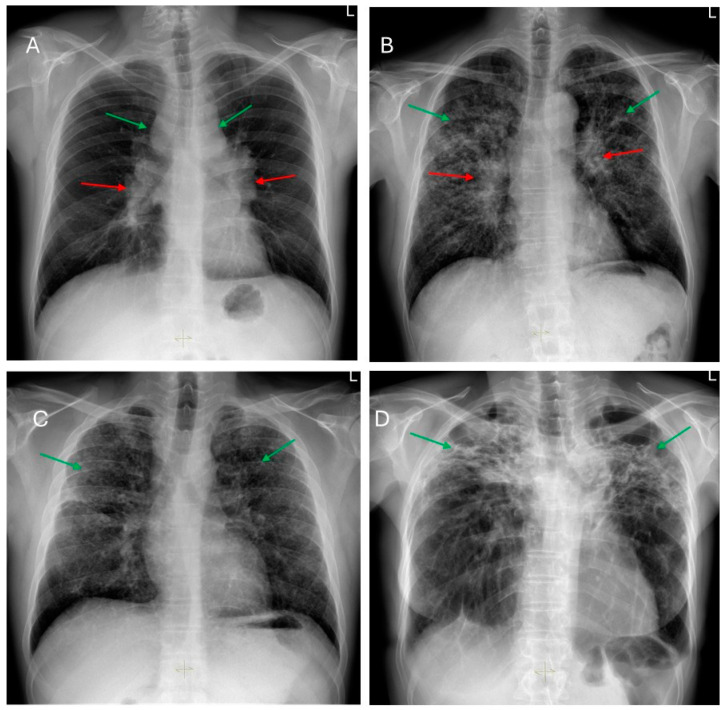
Frontal chest radiographs of patients with: (A)—Stage I—bilateral hilar (red arrows) and paratracheal (green arrows) lymph node enlargement. (B)—Stage II—diffused, multiple small nodular opacities predominantly located in the upper lung lobes (green arrows) and bilateral symmetric hilar lymph node enlargement (red arrows). (C)—Stage III—diffused, multiple small nodular opacities predominantly located in the upper lung lobes (green arrows). (D)—Stage IV—prominent upper zones fibrosis and volume loss (green arrows).
